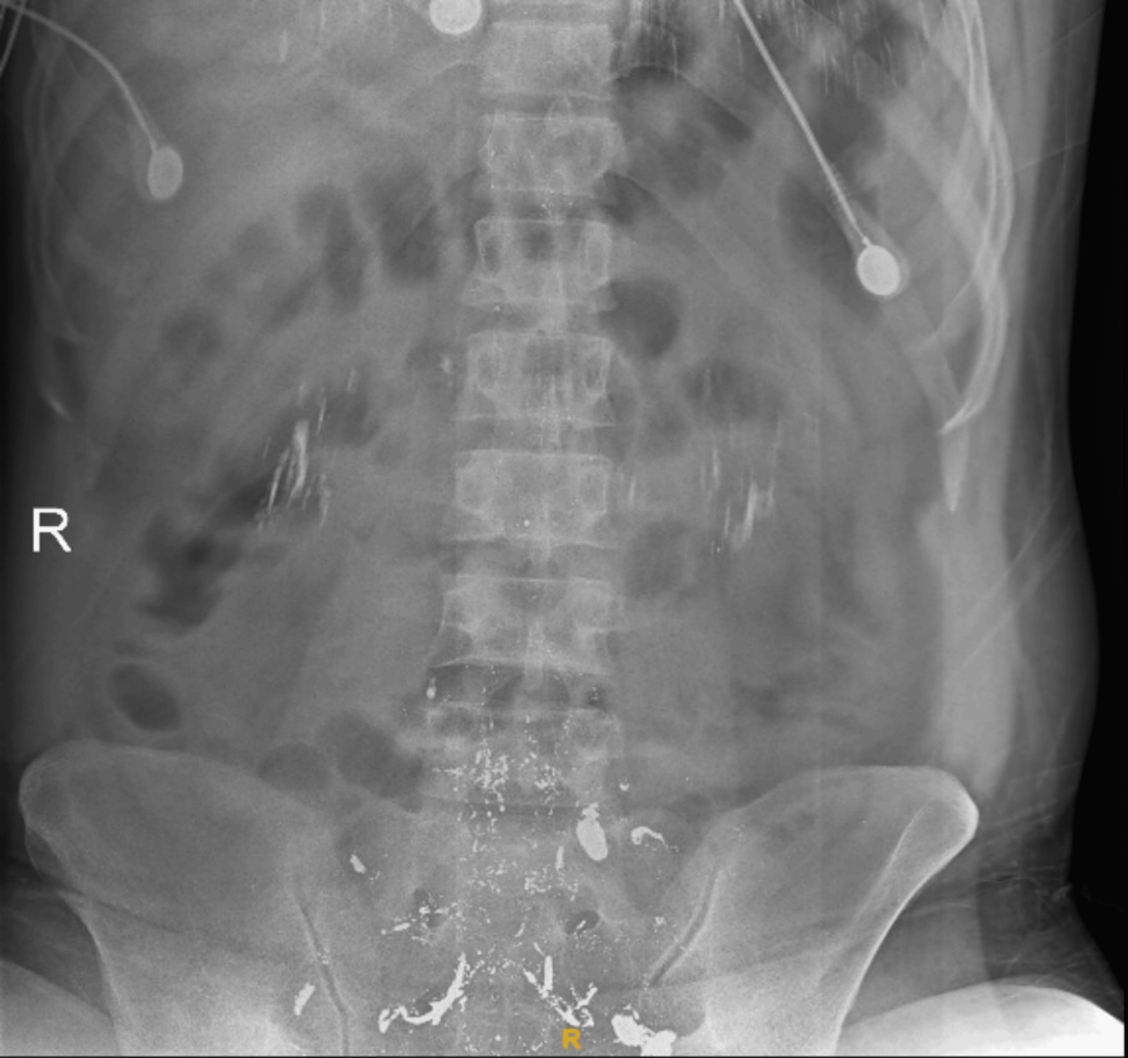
X-ray erect abdomen showing multiple variable sized radio-opacities in bilateral renal fossae and pelvis
